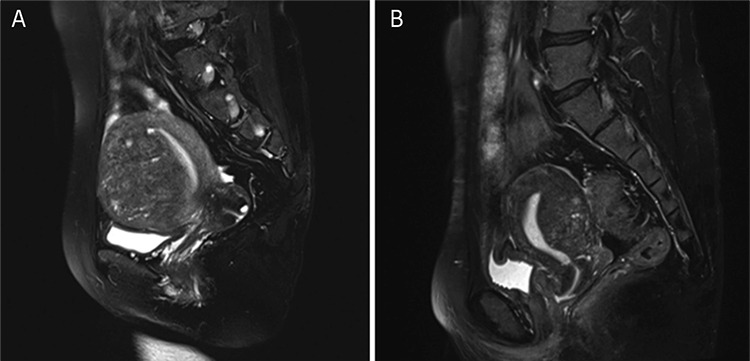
Magnetic resonance imaging imaging of adenomyosis The adenomyotic lesions in case one were located in the anterior uterine wall (A), and the lesions in case two were located in the posterior wall (B)
